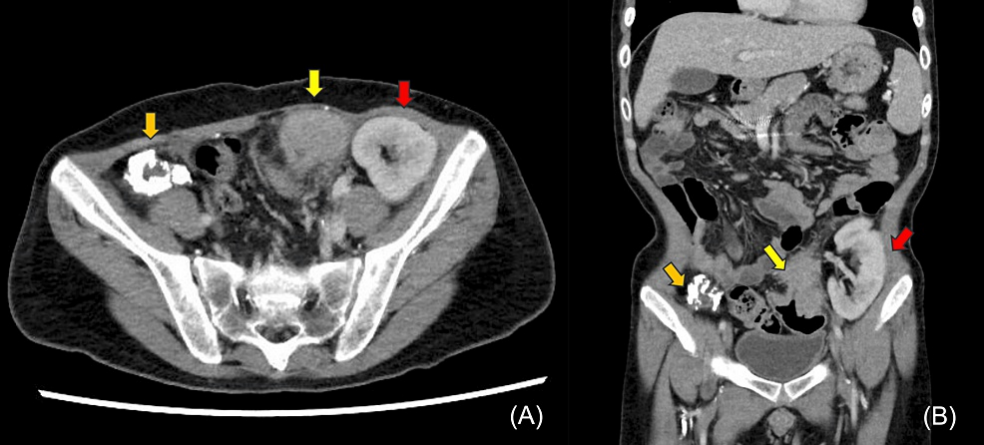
CT findings A CT scan revealed circumferential wall thickening with irregular surface and contrast enhancement on the sigmoid colon (yellow arrow) and near the left transplanted kidney (red arrow). The right transplanted kidney was atrophic and calcified (orange arrow). (A) Axial view. (B) Coronal view.
